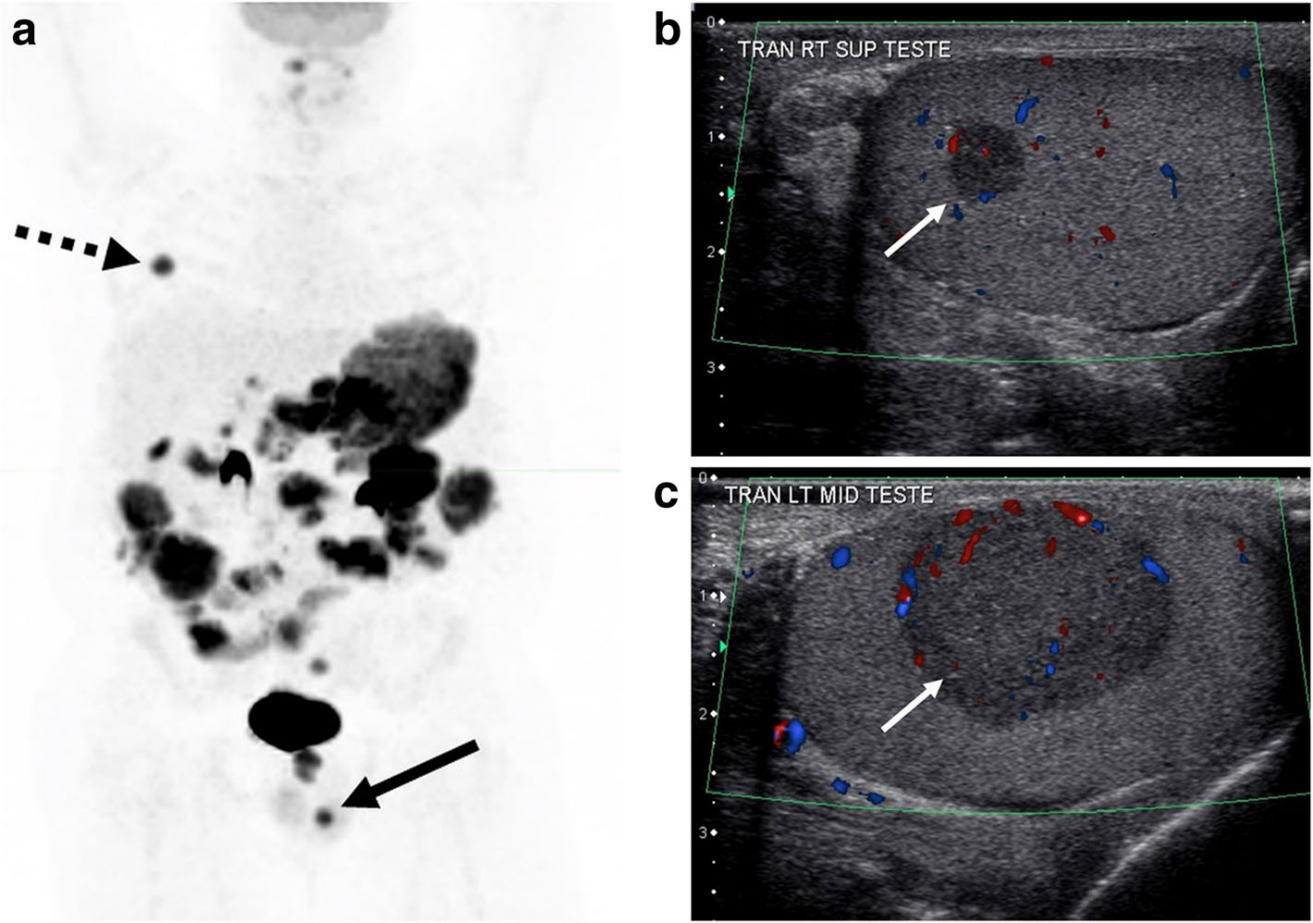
Bilateral testicular masses. A 39-year-old HIV-positive male with known Burkitt lymphoma. a Whole body planar PET image demonstrates widespread abdominal disease with a small focus of hypermetabolic activity within the region of the left testicle (solid arrow). Less intense, linear activity within the abdomen corresponds to physiologic bowel activity. Small focus of uptake in the right hemithorax (dashed arrow) corresponds to pleural disease. Sagittal color Doppler images through the (b) right and (c) left testicles demonstrate bilateral hypoechoic testicular masses, left larger than right (arrows)
